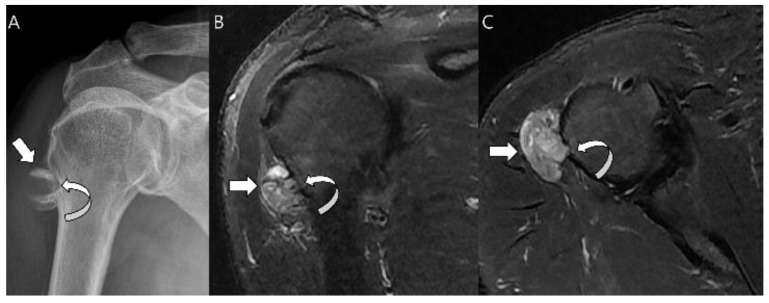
Periosteal chondrosarcoma grade 1 of the humerus in a 66-year-old man. (A) Radiograph shows a juxtacortical mass with Codman’s triangles (arrow) in the humerus. Note the associated cortical thinning (curved arrow). (B) Coronal and (C) axial T2-weighted images with fat suppression show a juxtacortical mass with high signal intensity and lobular margins (arrows). The mass has caused cortical erosion (curved arrow) but no evident marrow invasion.
